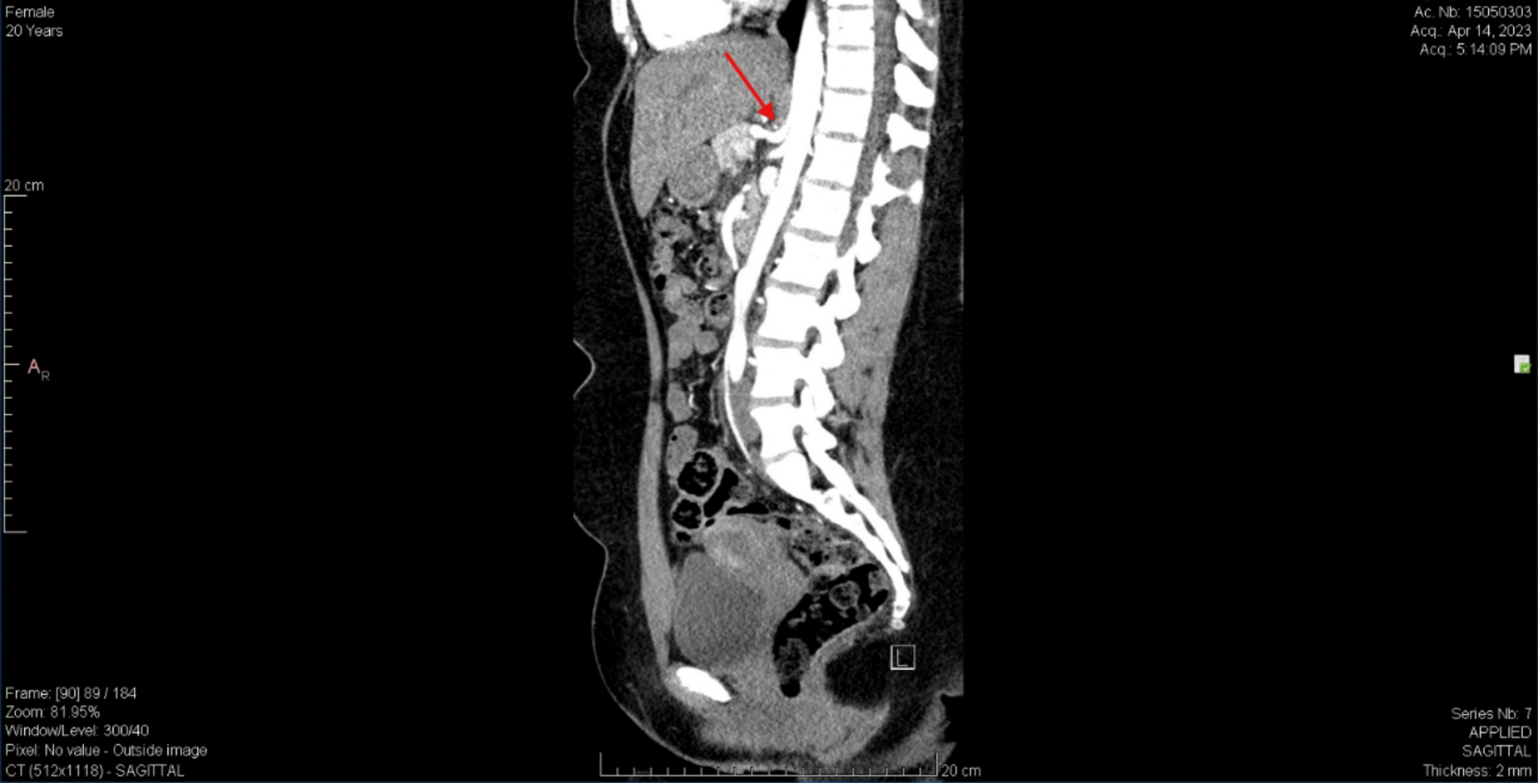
Sagittal CT scan of a patient with a low-riding median arcuate ligament abutting the celiac artery, causing slight vessel indentation, commonly called the “hook sign” due to the artery's acute angle, suggestive of MALS. Red arrow highlights the focal narrowing (or “hook sign”) of the proximal celiac artery consistent with extrinsic compression. CT: Computed tomography; MALS: Median arcuate ligament syndrome
